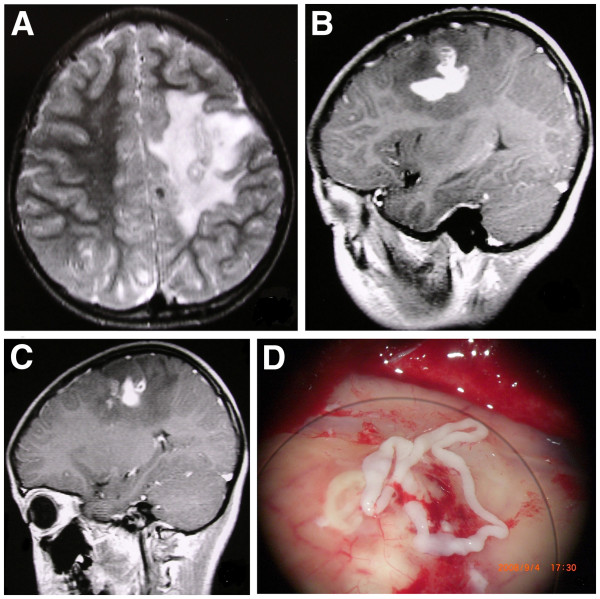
MR images for the case 12. A. The T2W image shows there is a serpiginous shape with inhomogeneous slight hyperintensity (Black arrow) in the left parietal lobe including mainly white matter area. Irregular large patchy edema can be seen in the perilesional parenchyma (White arrow) which is high signal on the T2W image. B. The enhanced T1W image shows a serpiginous tubular enhanced lesion with a diameter of 6.1 cm (which is the longest in the study). C. The enhanced T1W image from the MR follow-up after 7 months shows change in location and shape for the enhanced lesion. The lesion area was slightly moved medially and posteriorly, and appears as a comma shaped and ring enhancement. D. After the surgery, the worm was still alive, and its length was 13 cm.
